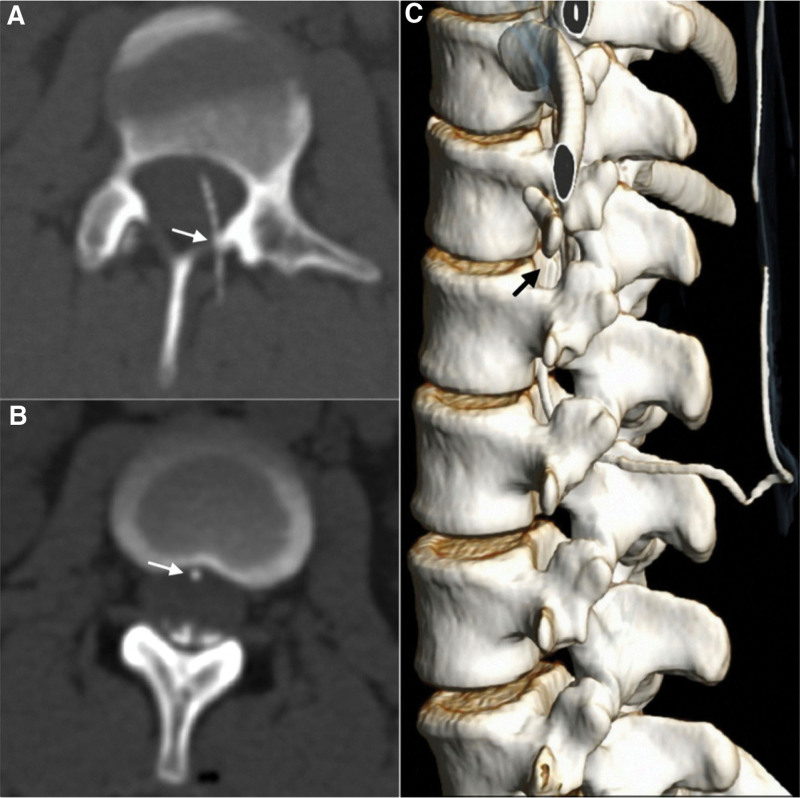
Computed tomographic myelography of thoracolumbar spine following instillation of contrast medium via the epidural catheter. (A) Axial image with MIP of the lumbar spine at L2 level showing the entry point (arrow) of the epidural catheter and the catheter traveling across the dorsal to the ventral portion of within the dural sac. (B) Axial image of the lumbar spine at L1-2 intervertebral disc level demonstrating the location of epidural catheter in the ventral site of the dural sac (arrow) and contrast medium collection in the dependent portion of the dural sac. (C) 3D reconstruction computed tomographic image demonstrated the virtual anatomy of the spine and the whole route of the epidural catheter in the subdural space. The tip of the epidural catheter was located at the T12-L1 disc level (black arrow). MIP = maximum intensity projection.
